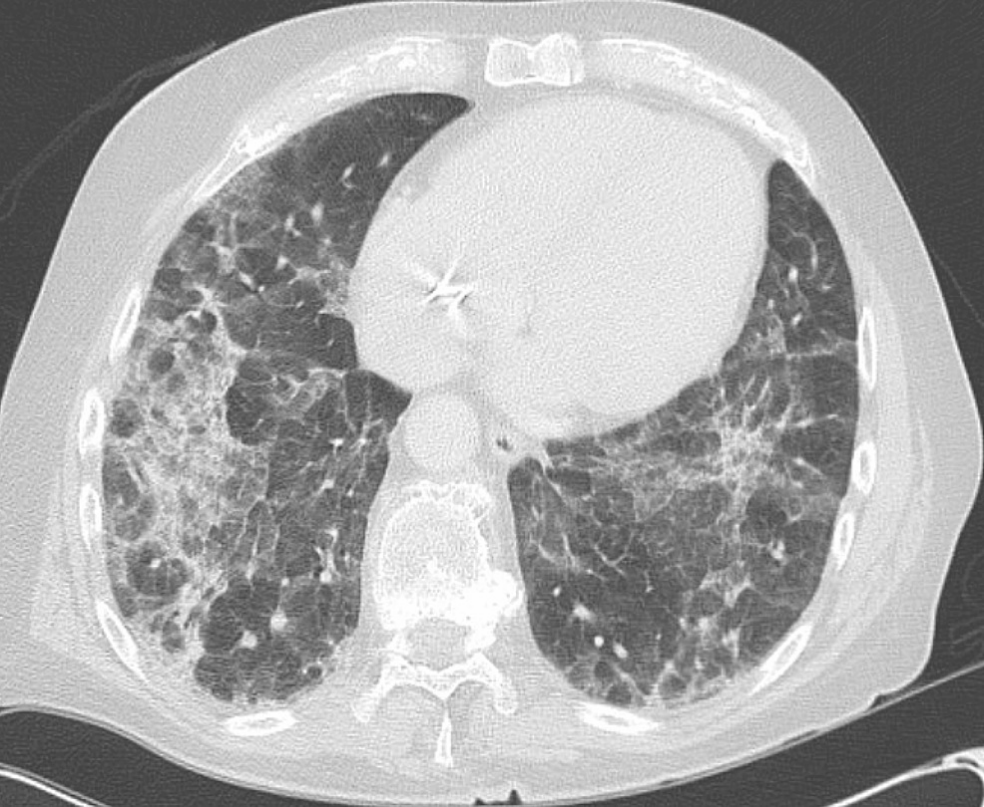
The patient's CT chest with IV contrast showing extensive ground-glass opacities throughout mid-lower lung zones.
